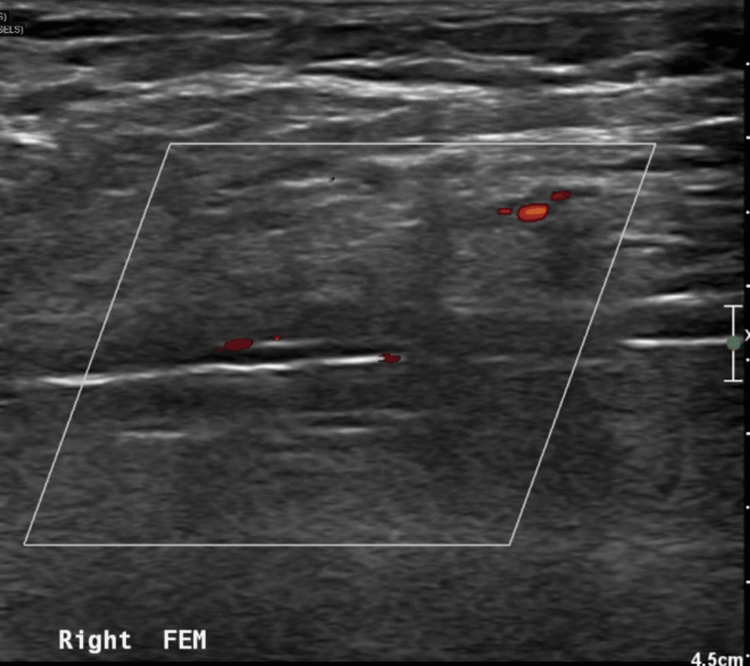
Longitudinal ultrasound image of the right common femoral artery with an inflated balloon in the lumen.
